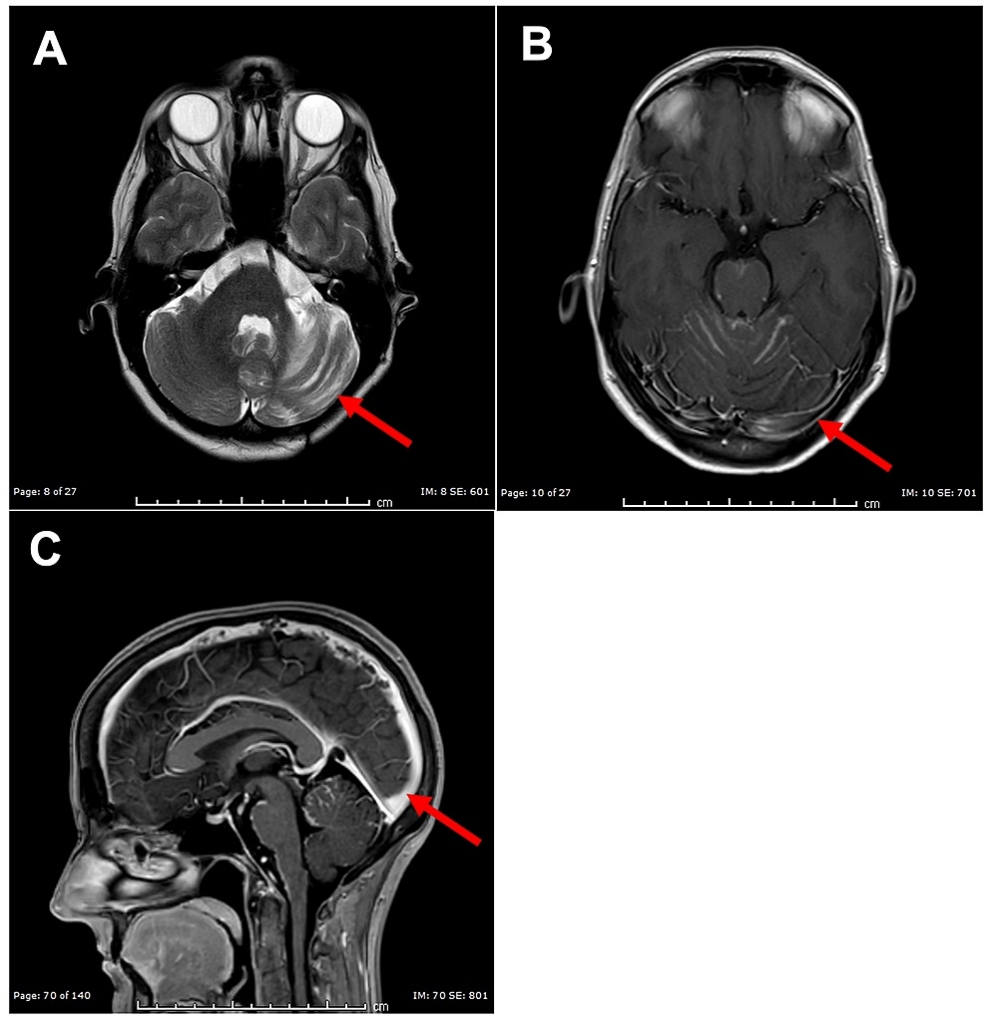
MR Images of Leptomeningeal Carcinomatosis (A) Axial T1 MR image with gadolinium contrast demonstrating onset of leptomeningeal carcinomatosis with stable cerebellar lesions (red arrow). (B) Axial T2 MR image demonstrating onset of leptomeningeal carcinomatosis with stable cerebellar lesions (red arrow). (C) Sagittal T1 MR image with gadolinium contrast demonstrating onset of leptomeningeal carcinomatosis with stable cerebellar lesions (red arrow).
